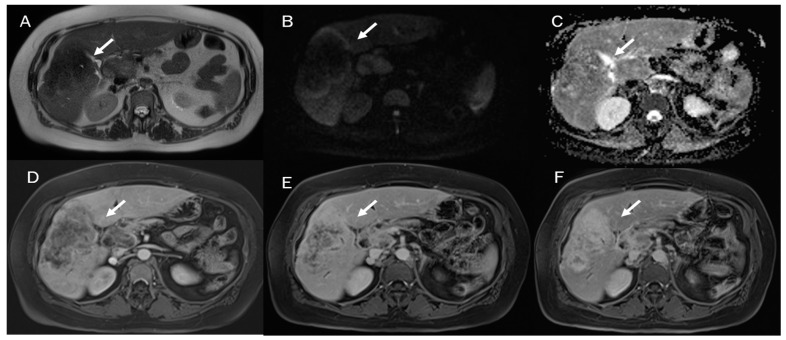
ICC MRI assessment. The lesion (arrow) shows hypointense signal in T2-W sequence (A) due to fibrotic tissue, with targetoid appearance in DWI (B) and ADC map (C) and progressive contrast enhancement during arterial (D), portal (E), and delay (F) phases of contrast study.
